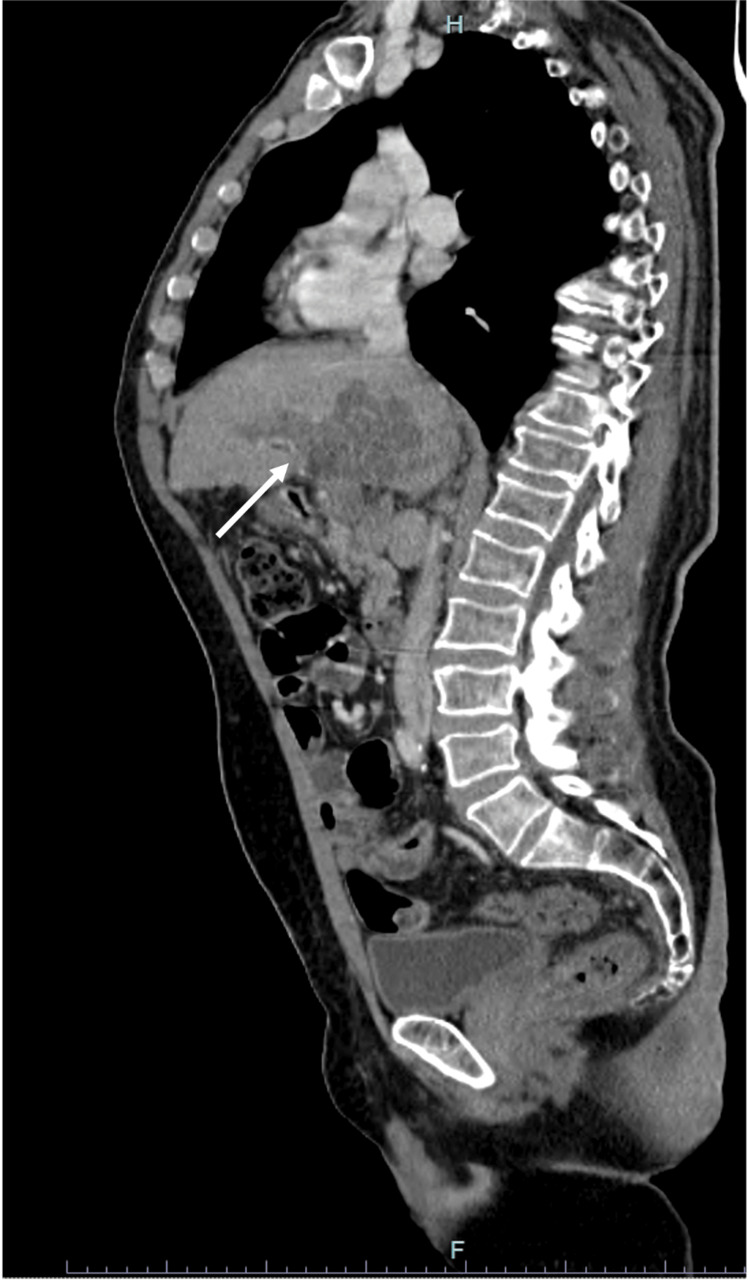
MRI three months after lenvatinib (stable disease)
